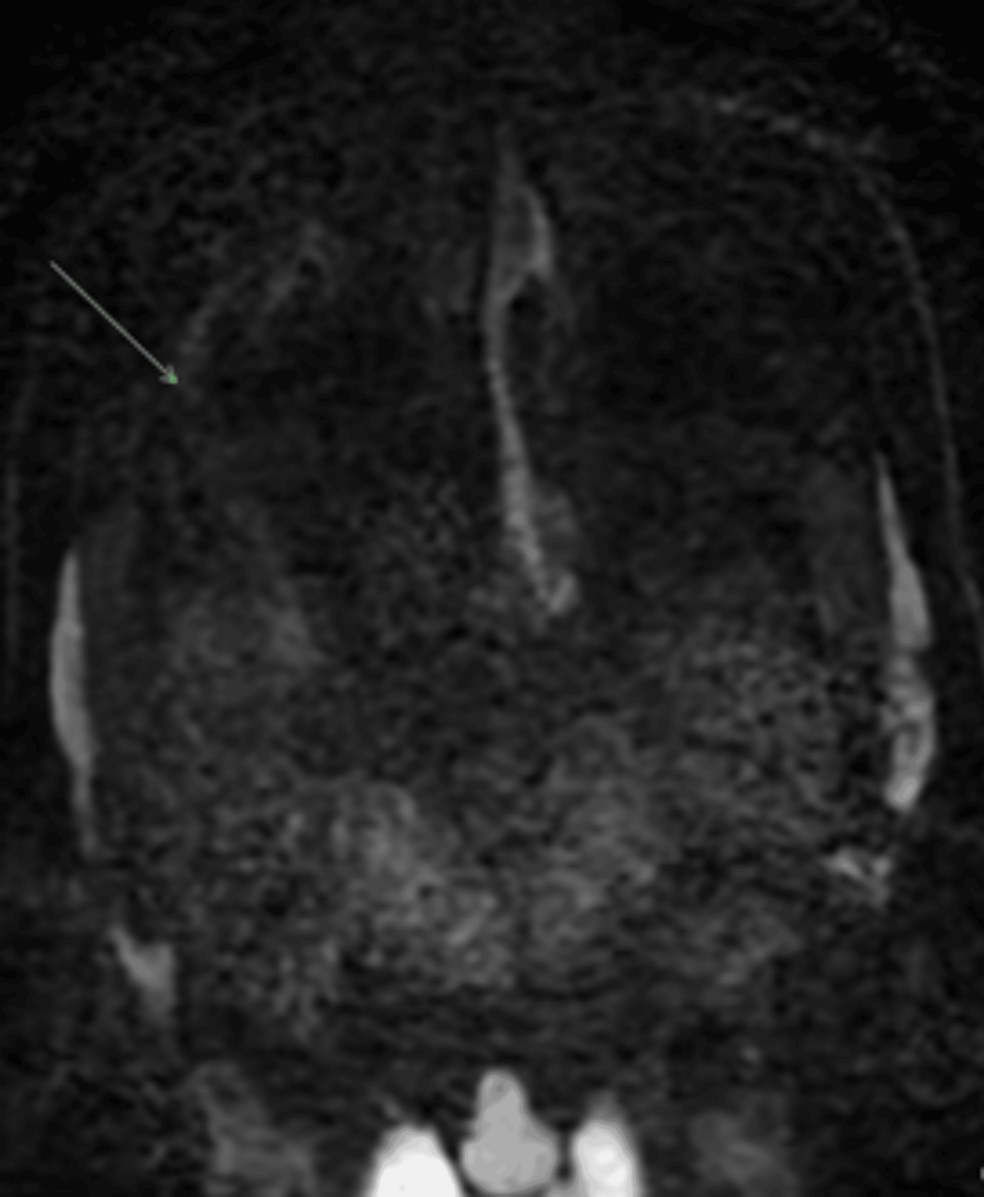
Post-chemotherapy diffusion weighted imaging (DWI) MRI August in 2023 shows normalisation of this area
